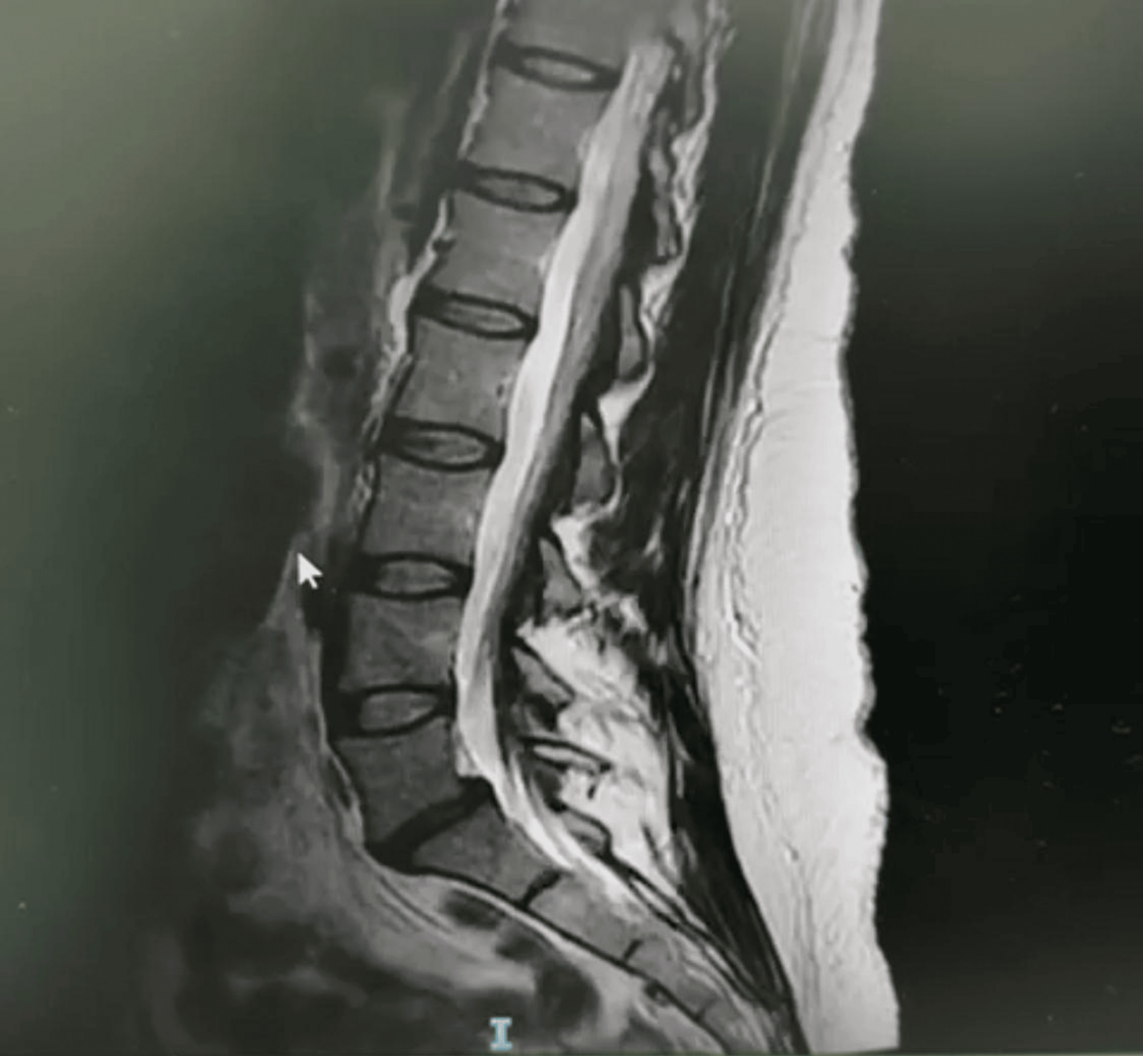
MRI findings showed no evidence of spinal cord infarction, acute ischemia, or compressive lesions
